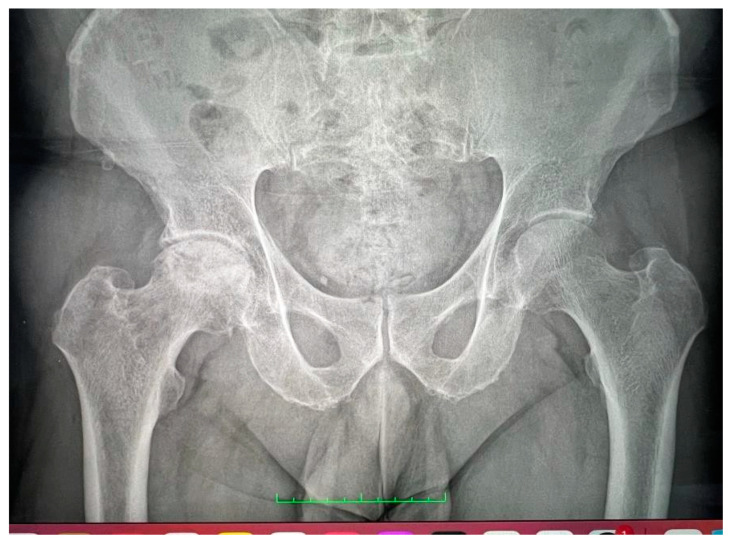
Bilateral X-ray with avascular necrosis.
